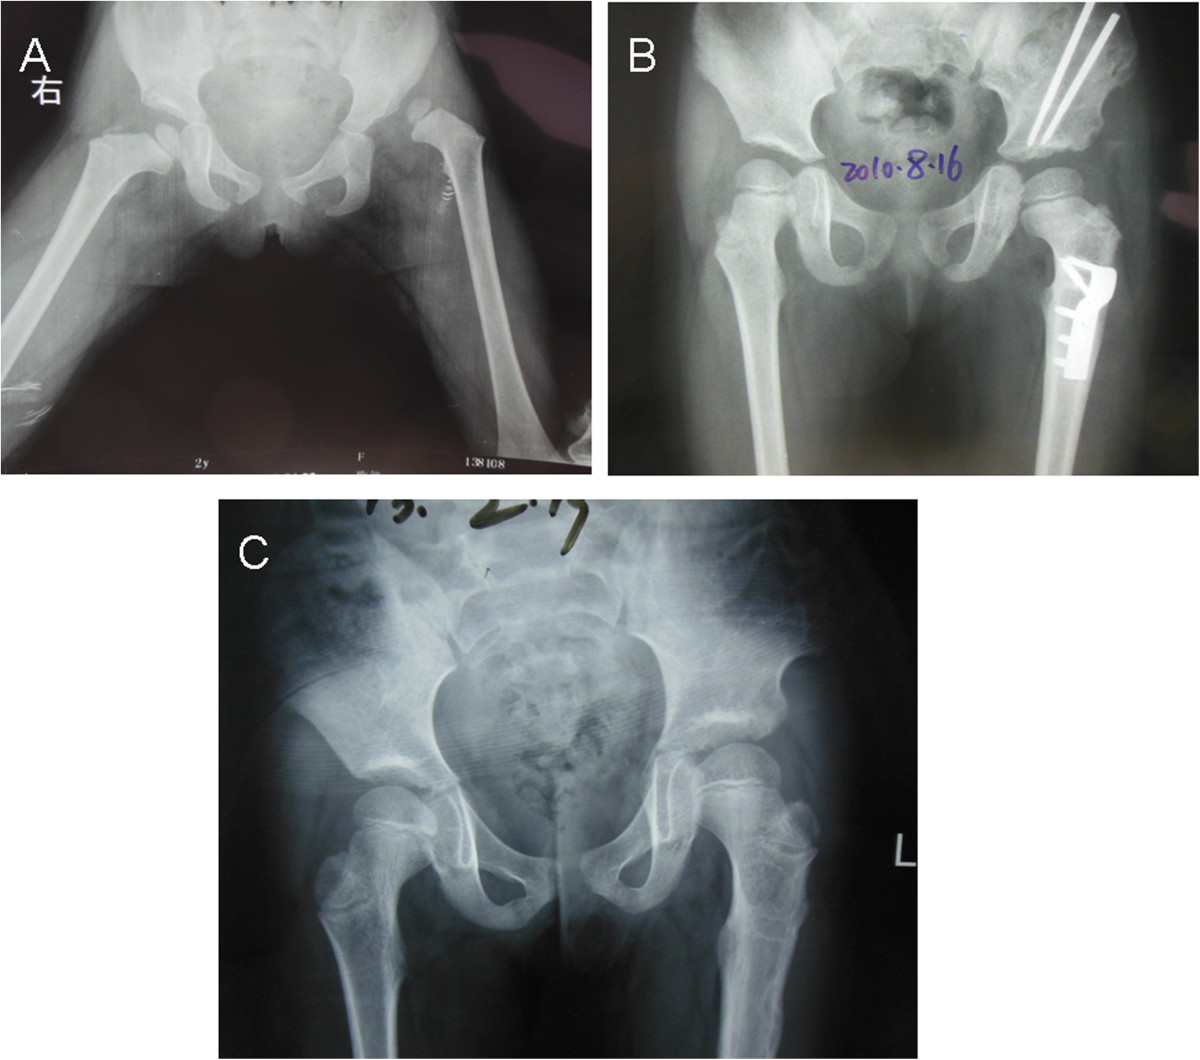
shows X-ray in a unilateral DDH, before therapy and at the follow up examination. A. Female patient aged 2.1 years with left DDH. Plain X-ray AP view. B. Plain X-ray AP view 1.6 years after one-stage operation, showing good containment of the femoral head. C. AP view 4.5 years postoperatively with excellent clinical and radiographic outcomes.
